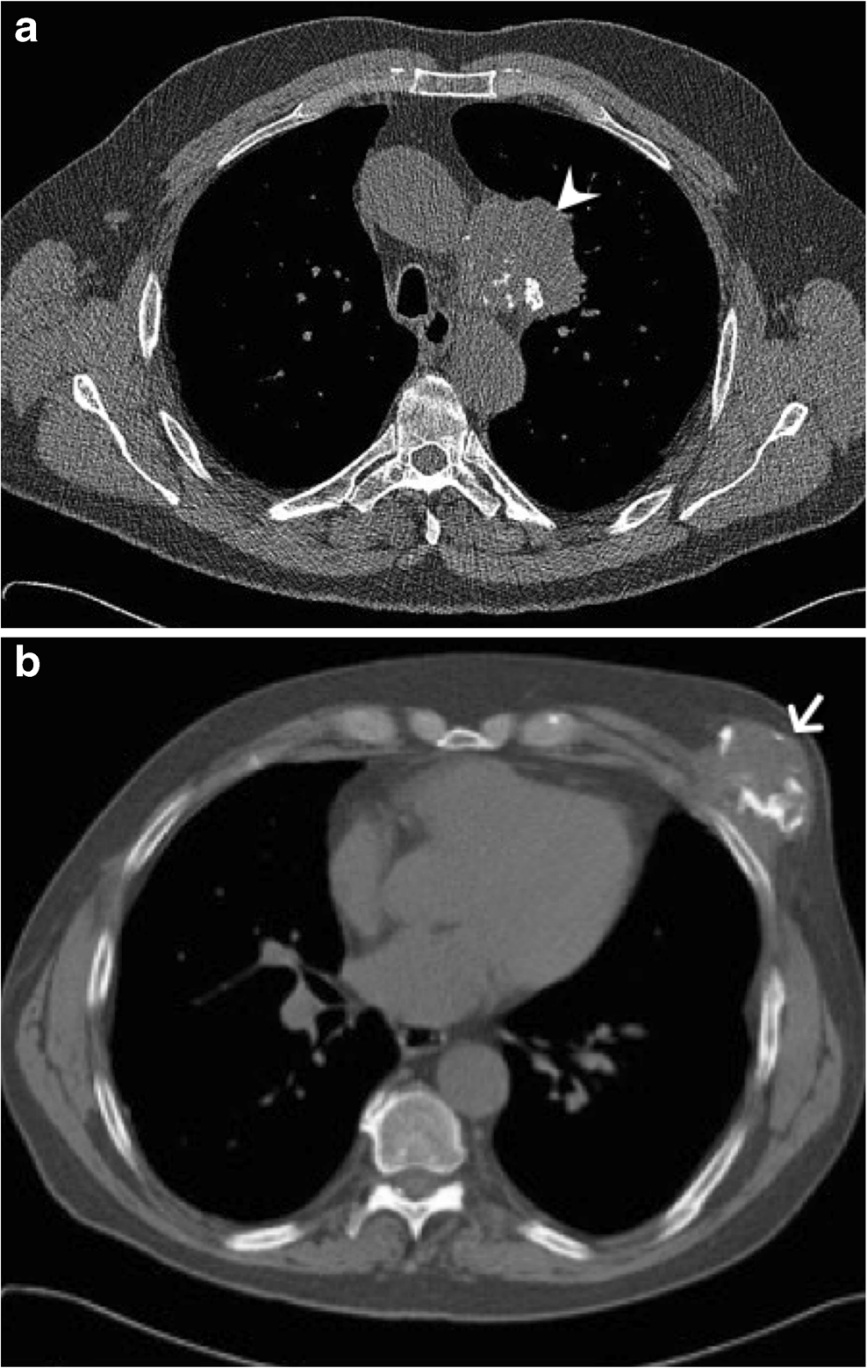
Metastatic calcified lung adenocarcinoma. (a, b) Two axial CT images of the thorax show calcified lung adenocarcinoma (arrowhead) (a) and calcified soft tissue chest wall metastasis (arrow) (b)
